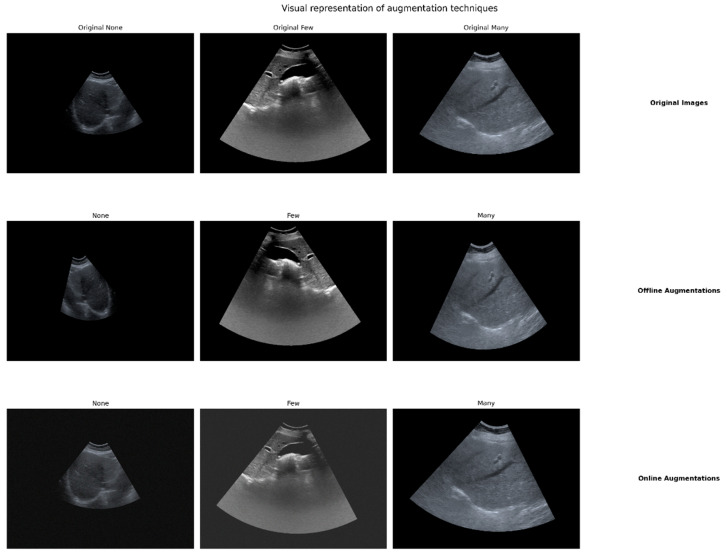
Visual representation of augmentation techniques applied to liver ultrasound images for hepatocyte ballooning detection. The figure shows original images (top row) and examples of offline (middle row) and online (bottom row) augmentations for each class (None, Few, and Many). Note the subtle variations introduced by each augmentation stage while preserving key diagnostic features.
